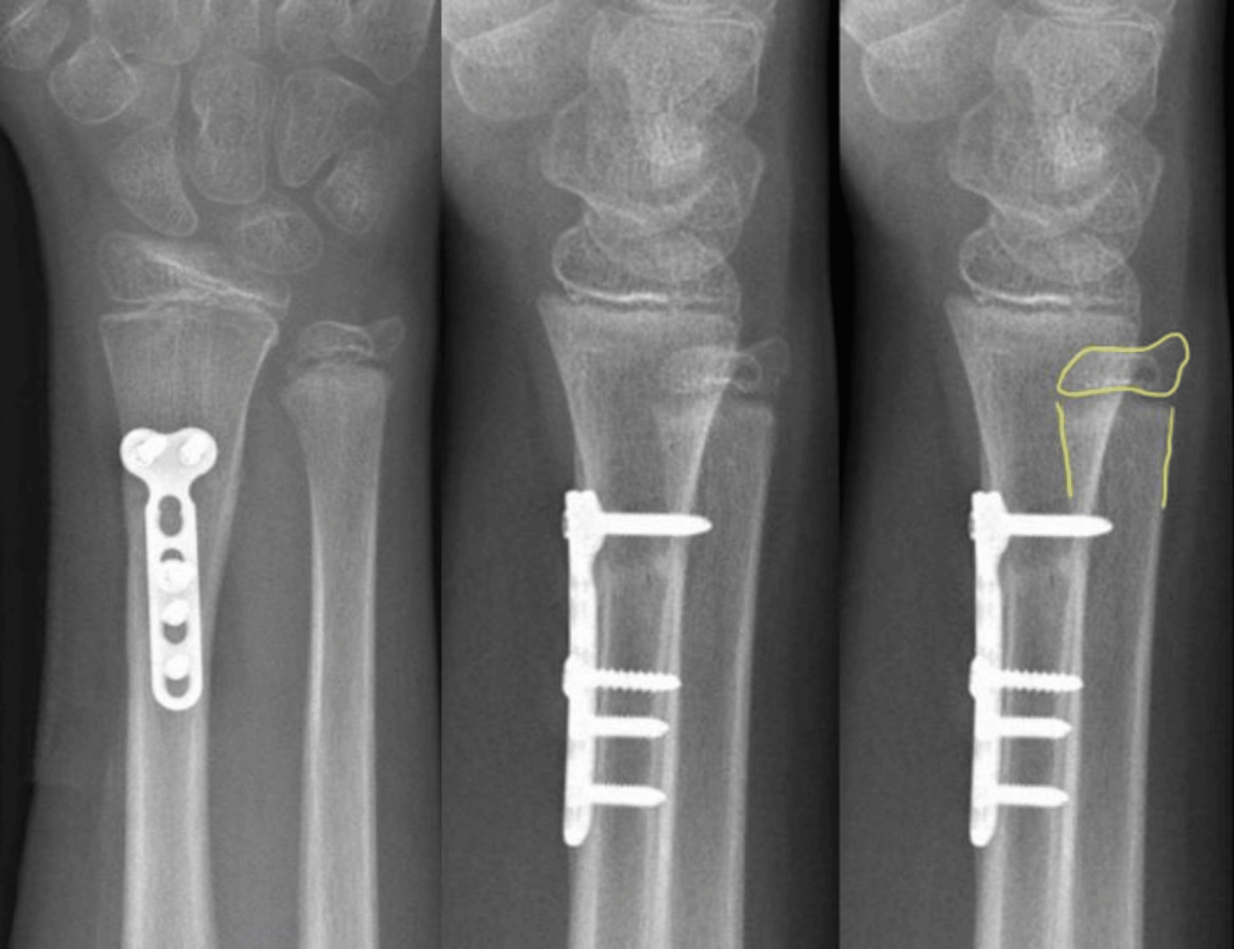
X-rays at six-month follow-up X-rays (anteroposterior and lateral view) with centered distal ulnar epiphysis. Yellow: epi-metaphyseal relation
